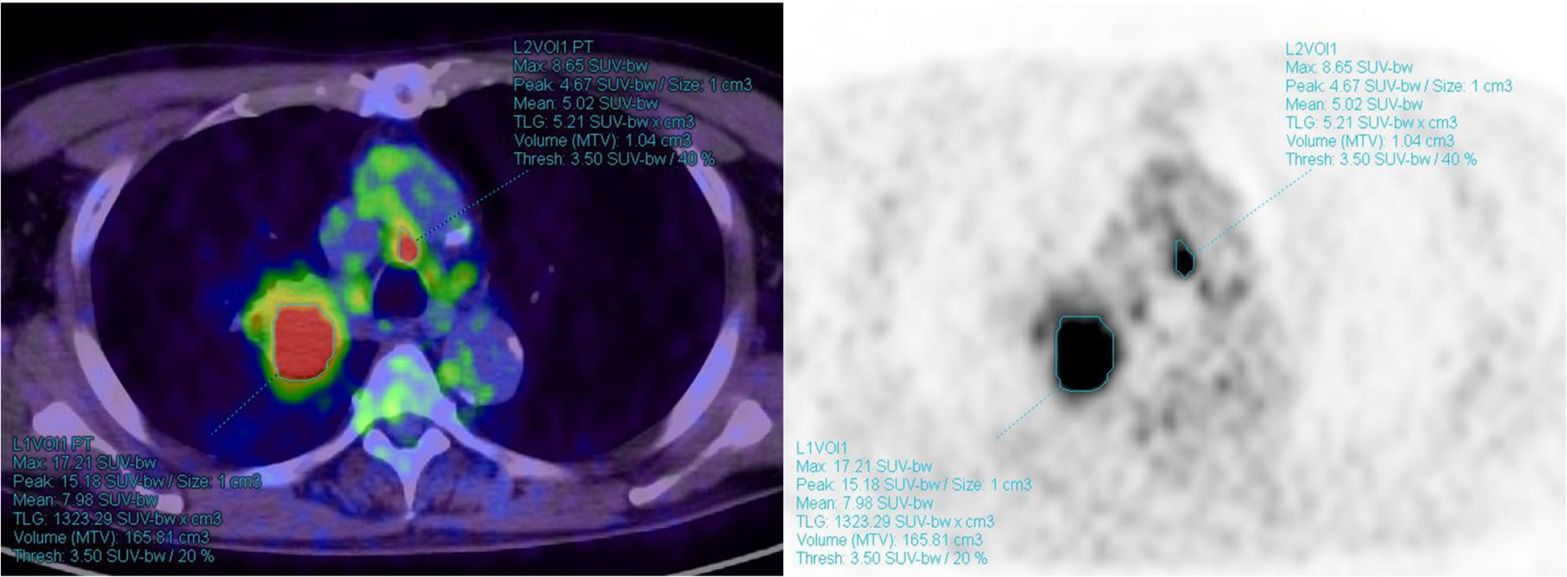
Representative example of the measurement of metabolic parameters on FDG-PET/CT for primary tumor and LNs. Primary tumor with an SUVmax of 17.21, MTV3.5 of 165.81 cm^3^, and TLG3.5 of 1323.29; #4R LN with an SUVmax of 8.65, MTV3.5 of 1.04 cm^3^, TLG3.5 of 5.21, and LPR of 0.5. In the pathological report, the LN was diagnosed as positive. FDG-PET/CT, fluorine-18-fluoro-2-deoxy-D-glucose-positron emission tomography; LN, lymph node; SUVmax, maximum standardized uptake value; MTV, metabolic tumor volume; TLG, total lesion glycolysis; LPR, lymph node-to-primary tumor ratio of SUVmax
